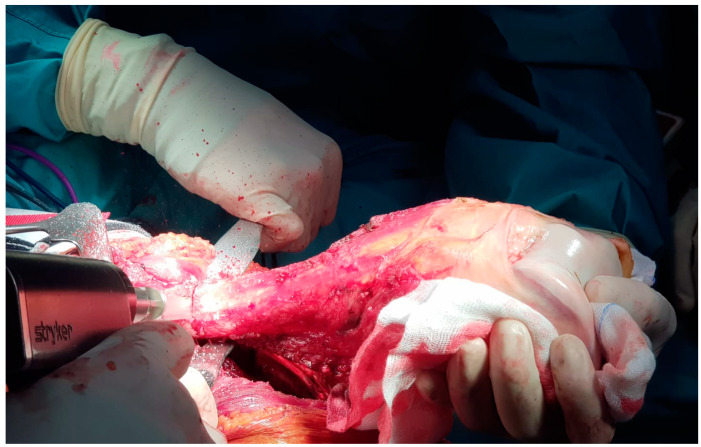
Intra-operative image of a distal femur resection. The distal end of the femur was isolated from the surrounding soft tissues, with the contextual sacrifice of insertion points for tendons and ligaments. The osteotomy line was identified using X-ray guidance and according to pre-operative planning. The bone shaft was exposed using Homan levers and cut using a motorized saw knife.
